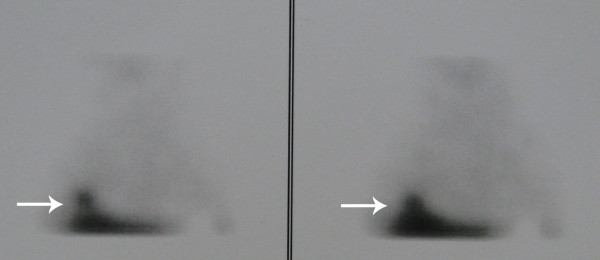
Technetium-99m-labeled octreotide acetate scintigraphy performed in single photon emission computed tomography mode. This was conducted 3 hours after injection of 15mCi technetium-99m-labeled octreotide acetate. The scan demonstrated a focal uptake in the lower lobe of our patient's right lung, just above his diaphragm, highly suggestive of an adrenocorticotropic hormone (ACTH)-producing bronchial tumor.
